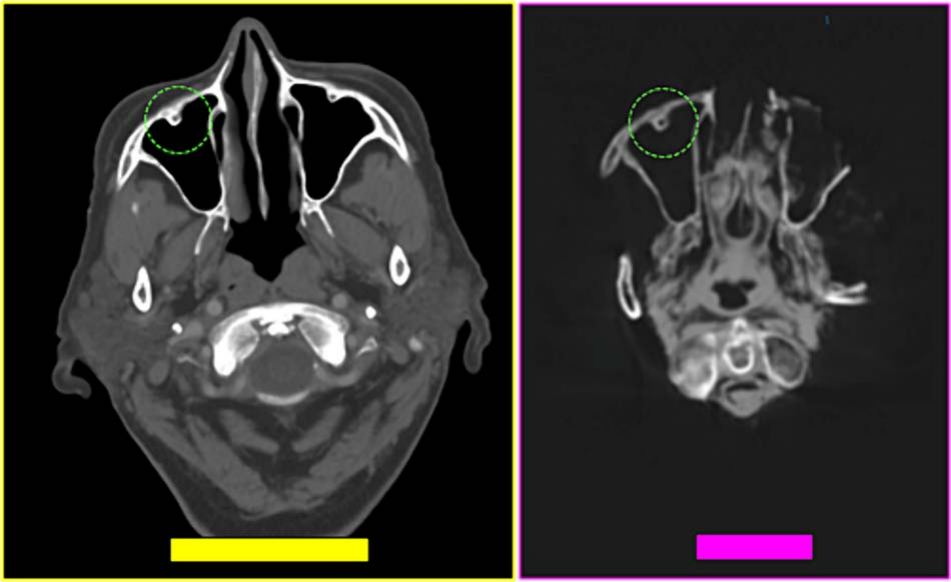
Coincident morphology of bone relief on the anterior wall of the right maxillary sinus (circumference) in the antemortem (left) and postmortem (right) CT scans.
